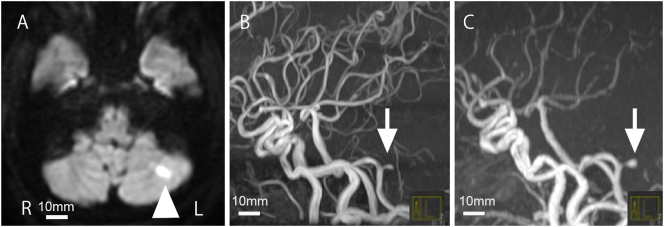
Preoperative brain MR images. A: A diffusion-weighted MR image showing acute cerebellar infarction in the left PICA territory (arrowhead). B–C: MR angiograms showing a saccular aneurysm at the origin of the PICA (B arrow), which increased in 2 weeks (C arrow).
